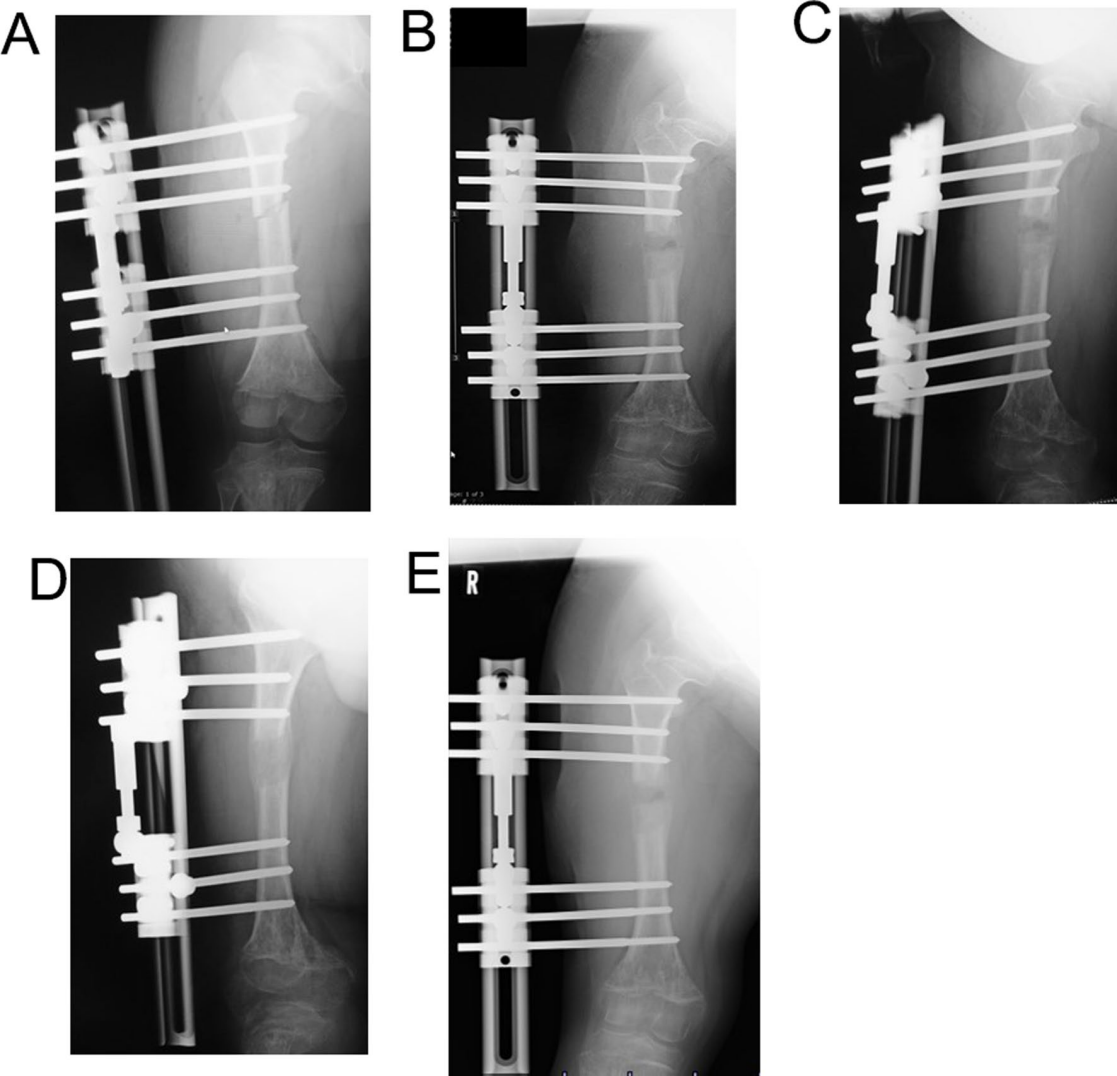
Postoperative X-ray of the femoral lengthening site that was treated using a unilateral external fixator in the previous hospital. A: Day 0. B: Day 30. C: Day 60. D: Day 120. E: Day 127
